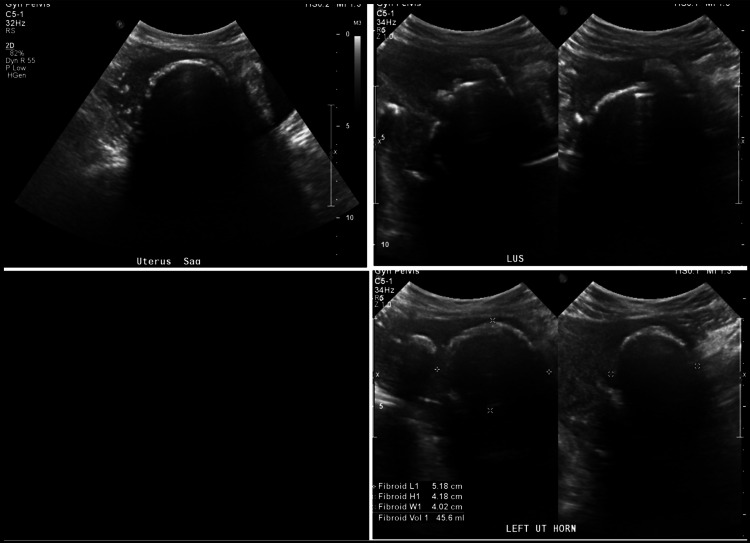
Multiple views of the pelvic US showing calcified fibroids measuring 5.2×4.2×4.0 cm and 5.1×5.1×5.9 cm. Does not appear significantly changed from prior CT of the abdomen/pelvis with contrast about one year prior. Similar in size to the pelvic US from about one year ago US: ultrasound
